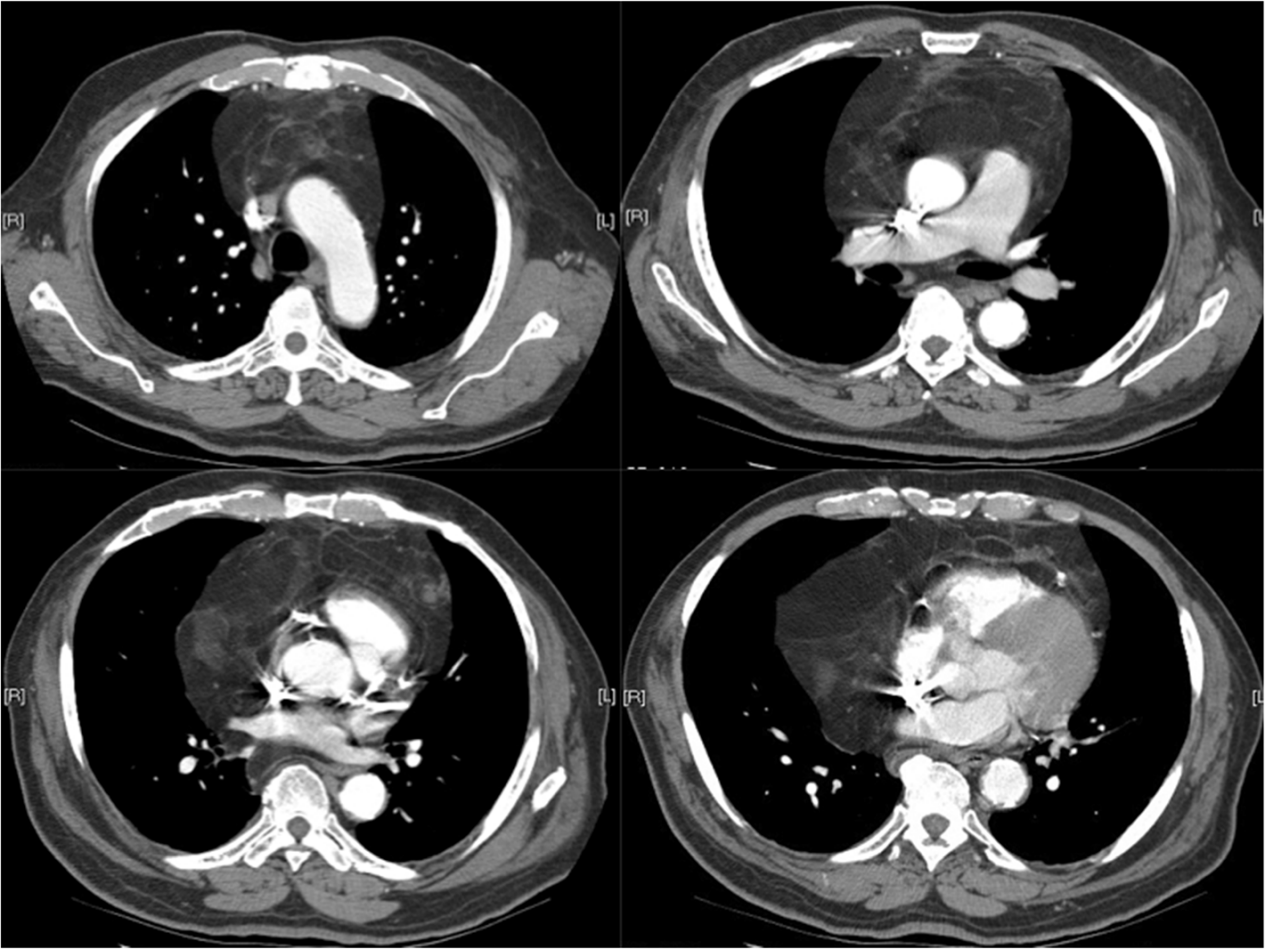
Preoperative computed tomography scan. Chest computed tomography scan at the level of pulmonary hilum (upper left image) demonstrates the multi-lobulated mass, well-defined, homogenous, low-attenuation mass in the anterior mediastinum. The other three images show lobulated mass with multiple calcific septations. The fat plane between the mass and the left pulmonary artery is focally obliterated.
